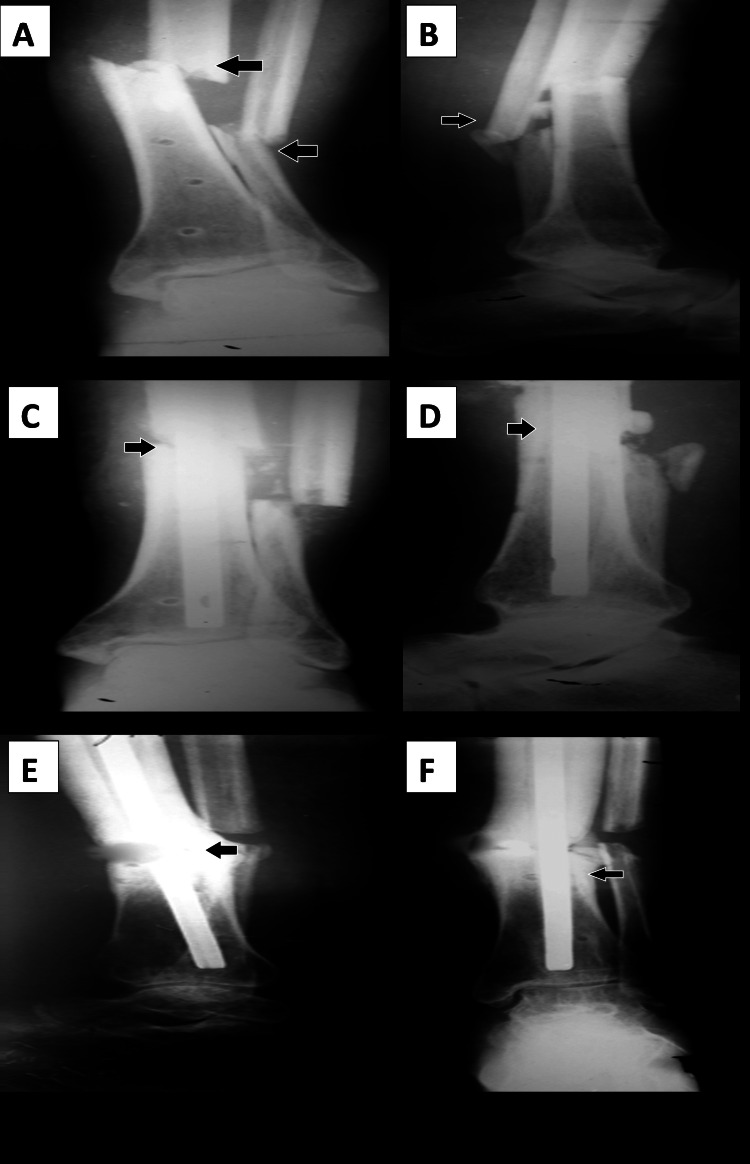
(A, B) Preoperative radiograph of a patient with infected nonunion of the tibia (arrows depicting nonunion tibia). (C, D) Postoperative radiograph (four weeks) of a patient with infected nonunion of the tibia (arrows depicting the alignment of nonunion tibia). (E, F) Postoperative radiograph (14 months) showing the bony union of a patient with infected nonunion of the tibia (arrows depicting the bony union of tibia).
